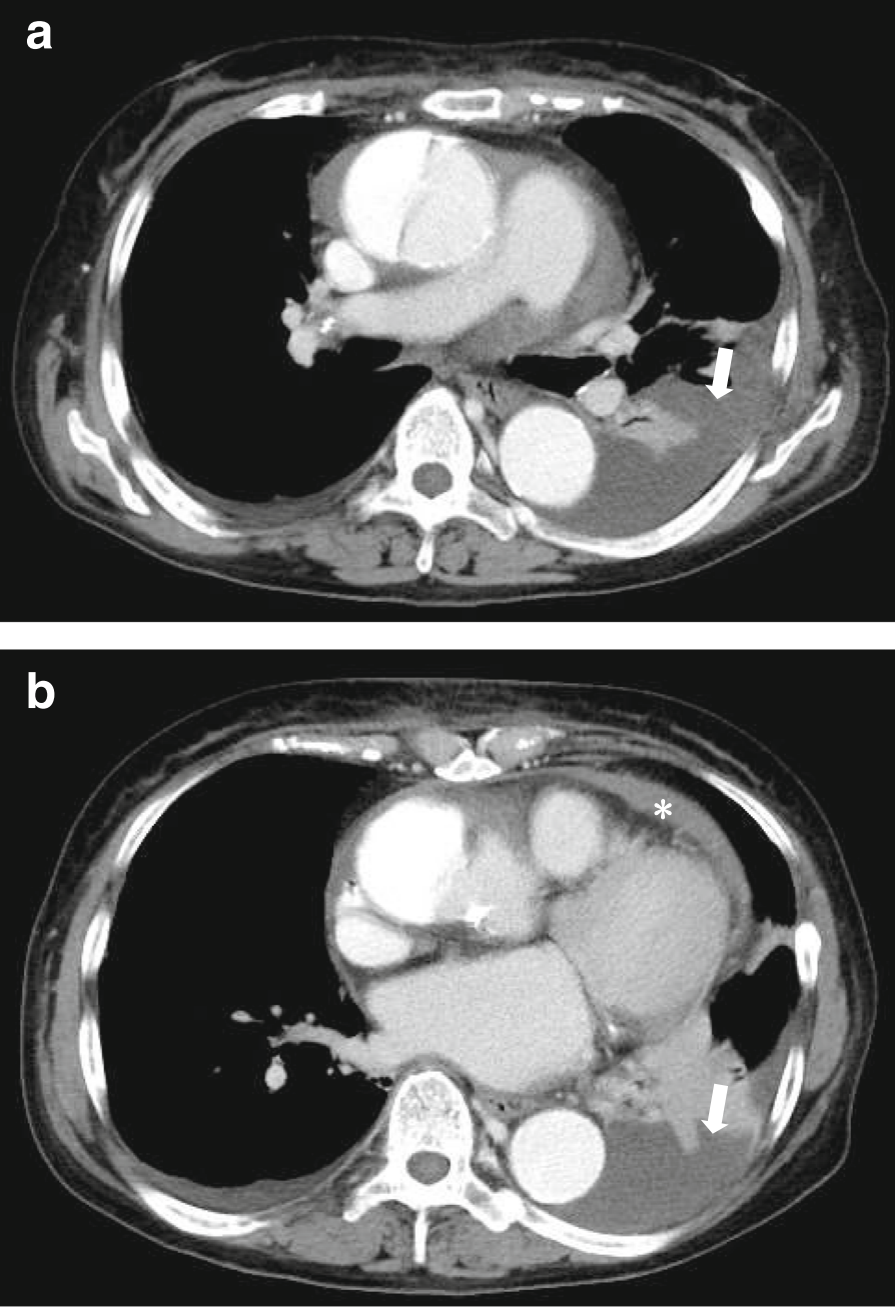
Enhanced computed tomography scans demonstrating aortic dissection and pleural effusion (arrow) (a) and pericardial effusion (asterisk) (b)
